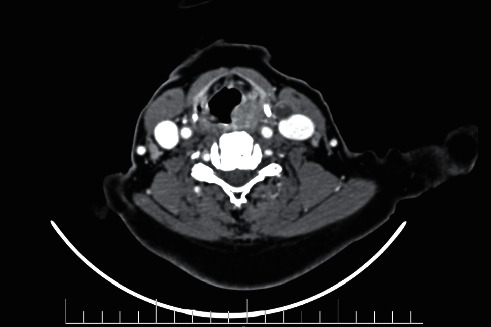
CT scan, axial view. There is an abnormal enhancing mass in the left pyriform sinus.
